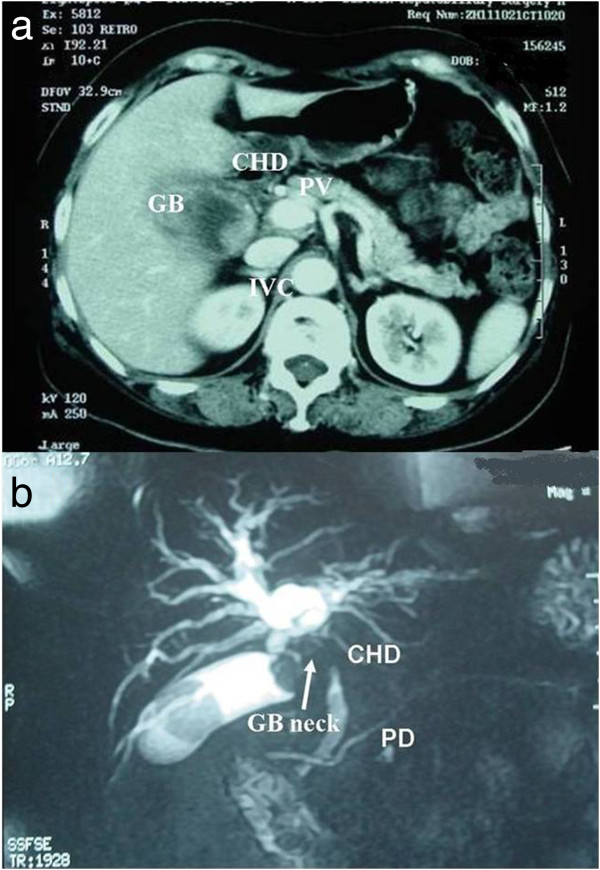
Typical imaging feature of gallbladder carcinoma involving the hepatic hilum. a. Enhanced CT shows gallbladder carcinoma with hepatic invasion. b. Preoperative MRCP shows that gallbladder carcinoma is located in the neck invading the hepatic hilum. The right hepatic artery and the common bile duct were involved by tumor in the surgery. We state that the subject of the photograph has given written informed consent by the patient to publication of the photograph. GB, gallbladder, CHD, common hepatic duct; IVC, inferior vena cava; PV, portal vein; PD, pancreatic duct.
